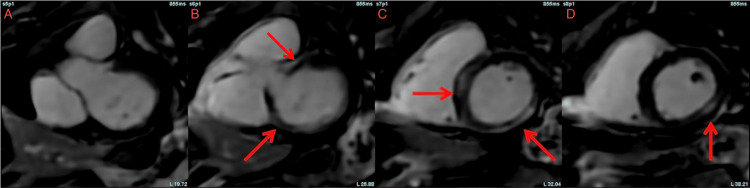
Cardiac MRI, short-axis stack of the left ventricle showing mid-myocardial (B) to epicardial (C and D) late gadolinium enhancement along the basilar lateral wall and basilar inferoseptal wall. The enhancement is consistent with T2 mapping for inflammation with a similar non-vascular distribution.
